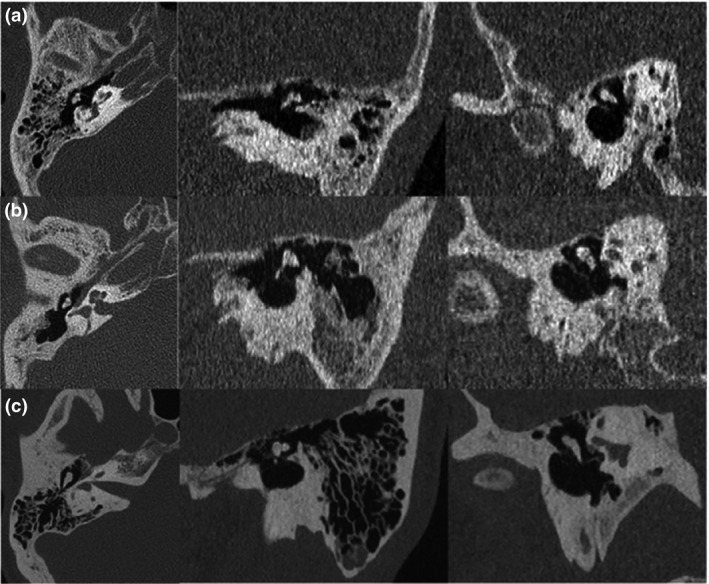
The image quality of temporal bone CT images produced with multiplanar reconstruction using different generations of DSCT scanners, shows the third generation scanners can produce better image quality using lower current to reduce effective dose: (A) first generation images using 180 mAs with ED of 0.67 mSv, (B) second generation slice using 138 mAs with ED of 0.41 mSv, (C) third generation axial slice with 103 mAs and ED of 0.24 mSv.^42^ Permission was obtained to reproduce these images.
